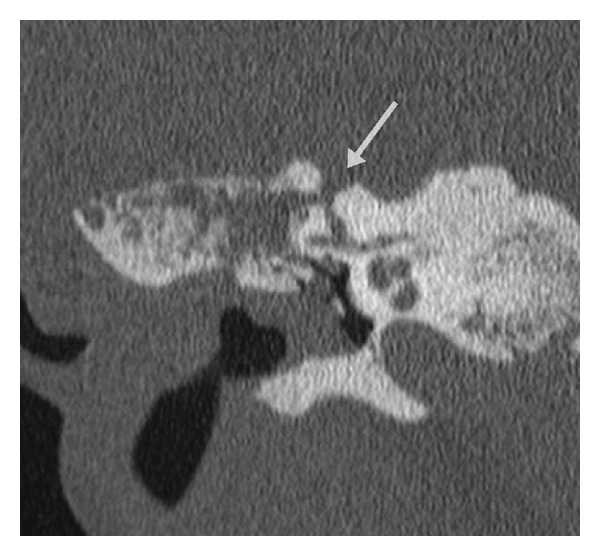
CT imaging of the patient in Case 2. Coronal section demonstrates a right temporal bone fracture (arrow) traversing the bony superior semicircular canal.
